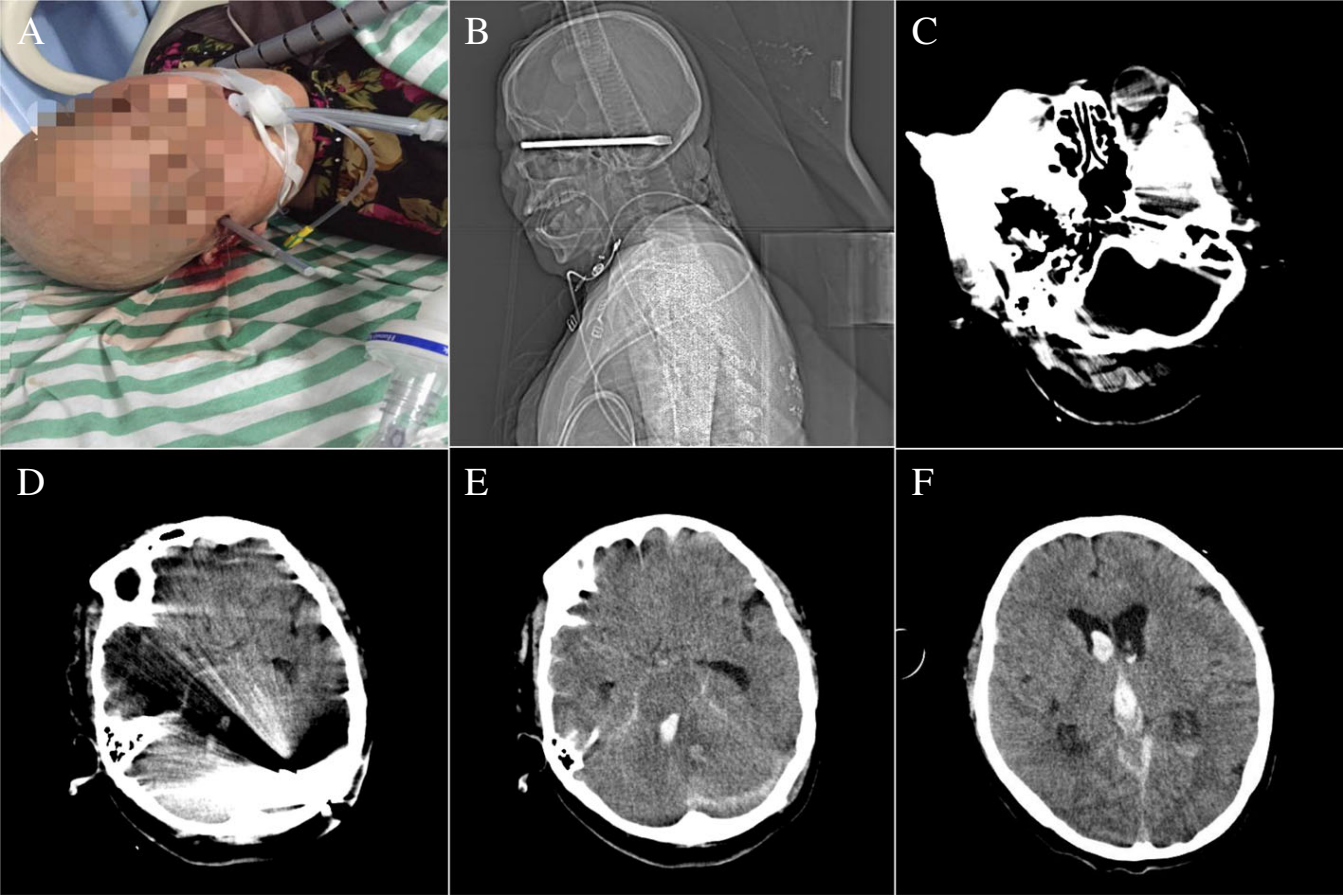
a. Clinical picture showing a strewdriver penetrating the right cheekbone. b. Computed tomogram (CT) of brain showing the metallic foreign body with metal artefacts. c-d. CT of head-brain window showing a metallic foreign body passed through the right cheekbone to posterior cranial fossa. e-f. CT scan showing left cerebellar hematoma and subarachnoid hemorrhage
